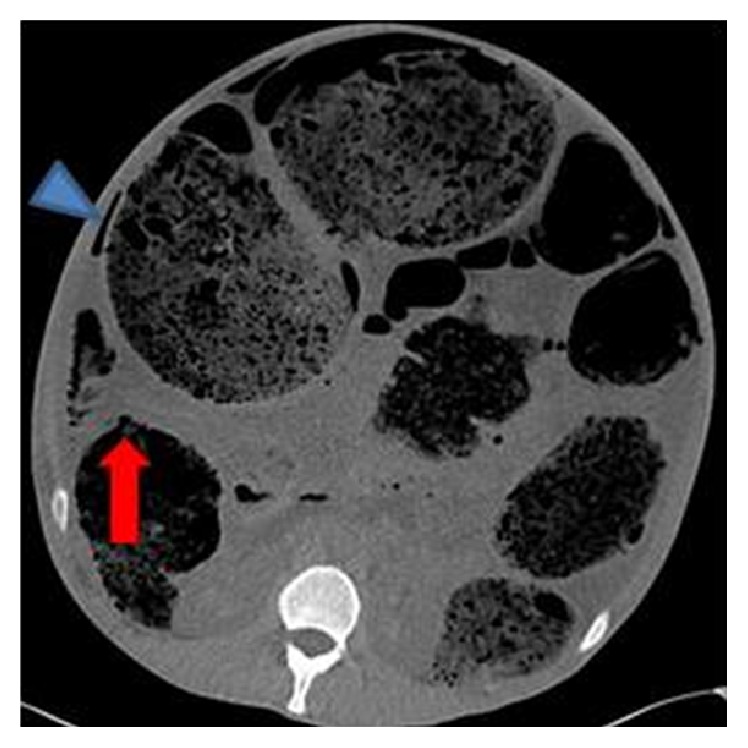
Axial CT images on soft tissue windows showing the anterior ascending colon perforation site (arrow). The ascending colon has collapsed a little relative to the distal bowel. No definite intramural gas in the wall. There is right-side pneumoperitoneum (arrowhead).
